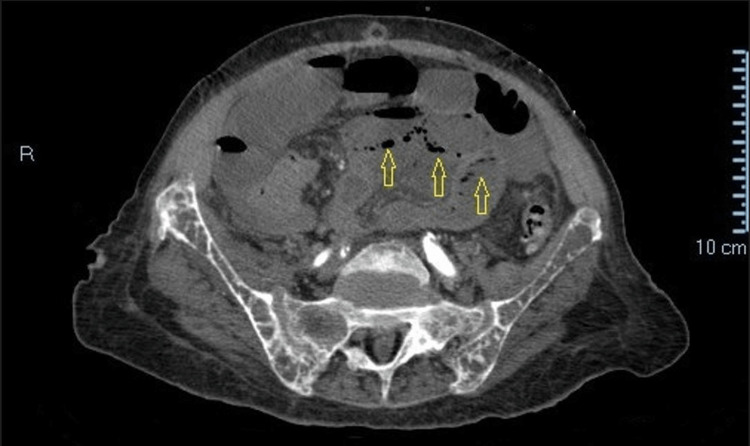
A contrast-enhanced CT scan shows diffuse intestinal pneumatosis (yellow arrows)
